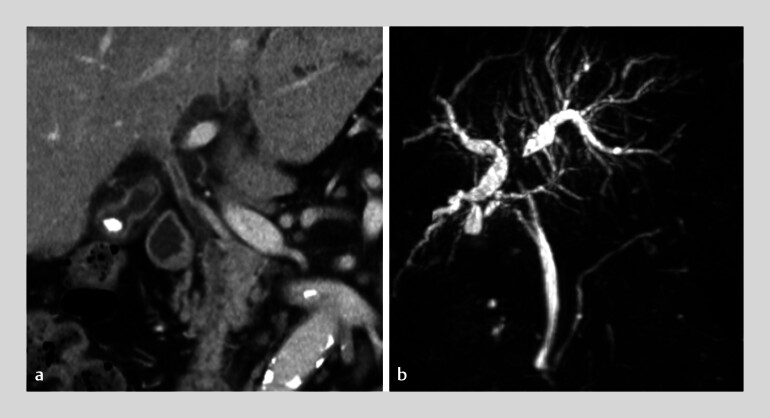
Computed tomography (CT) and magnetic resonance cholangiopancreatography (MRCP) images of malignant hilar biliary obstruction.
